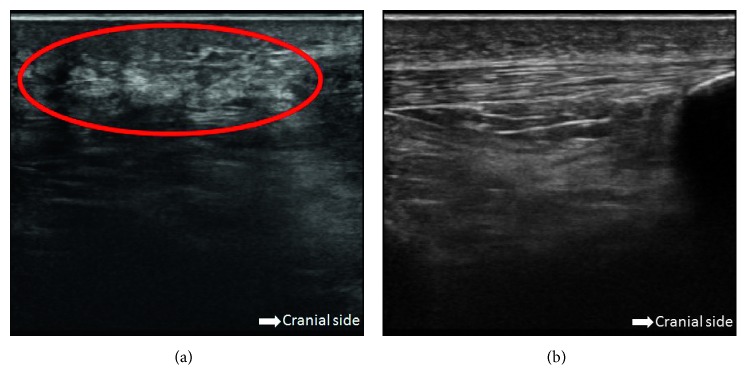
Ultrasound examination showed a loose patellar tendon (within red circle) (a) compared with the contralateral side (b). The right is the cranial side, and the patella can be seen on the far right in (b).
